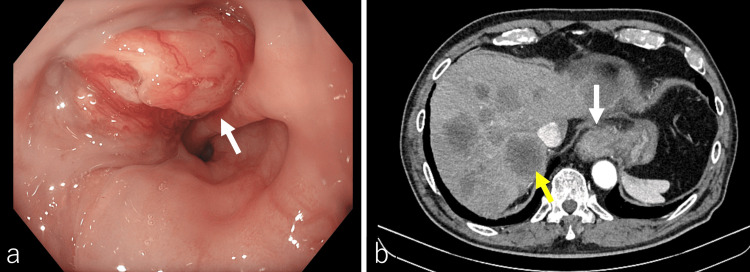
Initial endoscopy and abdominal contrast-enhanced CT Upper gastrointestinal endoscopy revealed a 3-cm tumor at the esophagogastric junction (a, white arrow). Contrast-enhanced CT demonstrated a mass at the esophagogastric junction (b, white arrow) and multiple liver metastases (b, yellow arrow).
